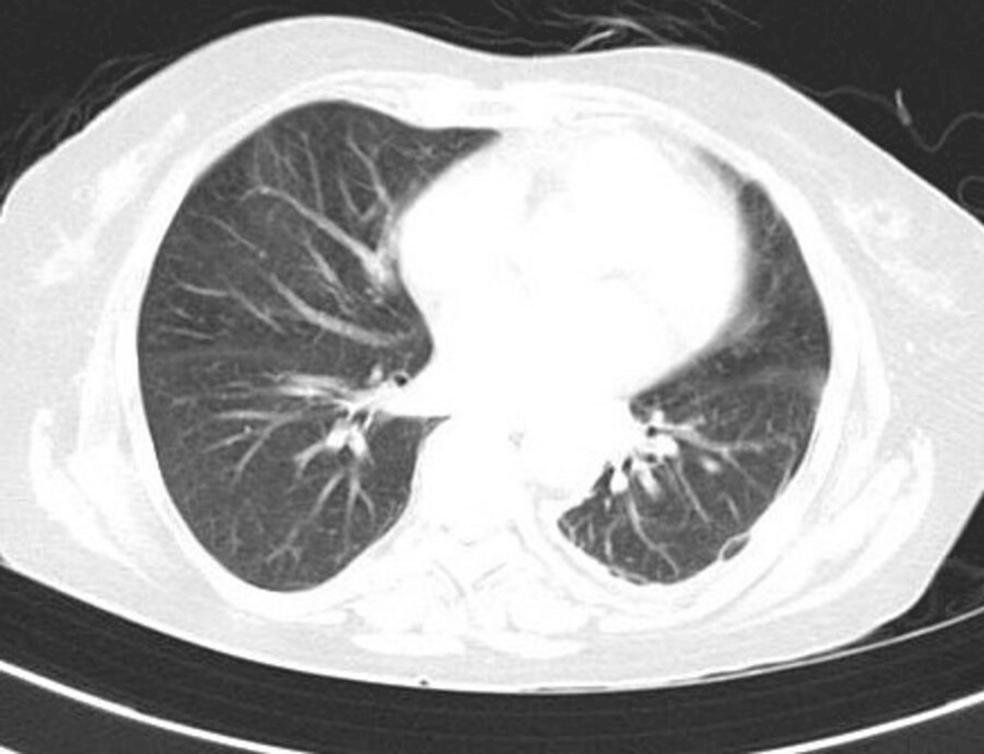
HRCT thorax of a patient after one month of discharge from the hospital. HCRT: high-resolution computed tomography.
